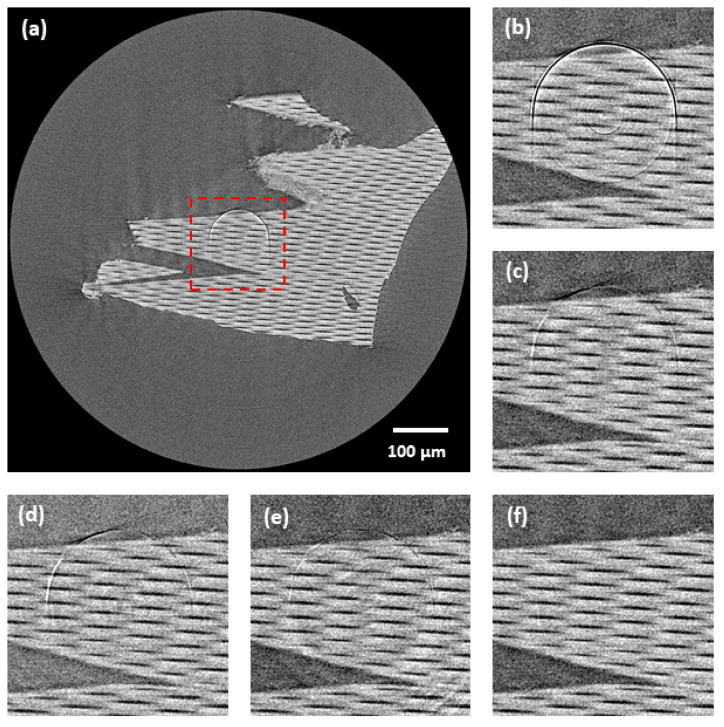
Comparison of tomograms after ring artifacts reduction by tested methods—real computed tomography (CT) data of glass capillary array acquired with a CCD-based camera: (a) original tomogram (without any correction)—red labelled area marks central area visualized in following images; (b) original; (c) M1; (d) M2; (e) M3; (f) proposed. For visualization, the same contrast setting was used for all the images.
